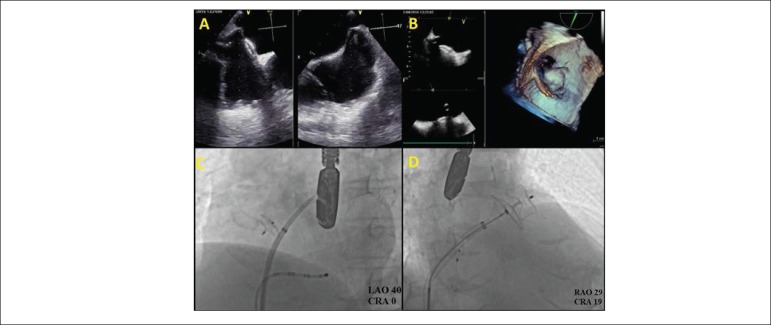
a) Transesophageal echocardiography image of the transseptal puncture needle tenting the inferoposterior site of the interatrial septum and atrial septal defect closure device, b) Tridimensional transesophageal echocardiography guidewire image after the transseptal puncture, c) Fluoroscopic image of the transseptal puncture on the inferoposterior site of the interatrial septum and atrial septal defect closure device, d) Fluoroscopic image of the device before being released.
